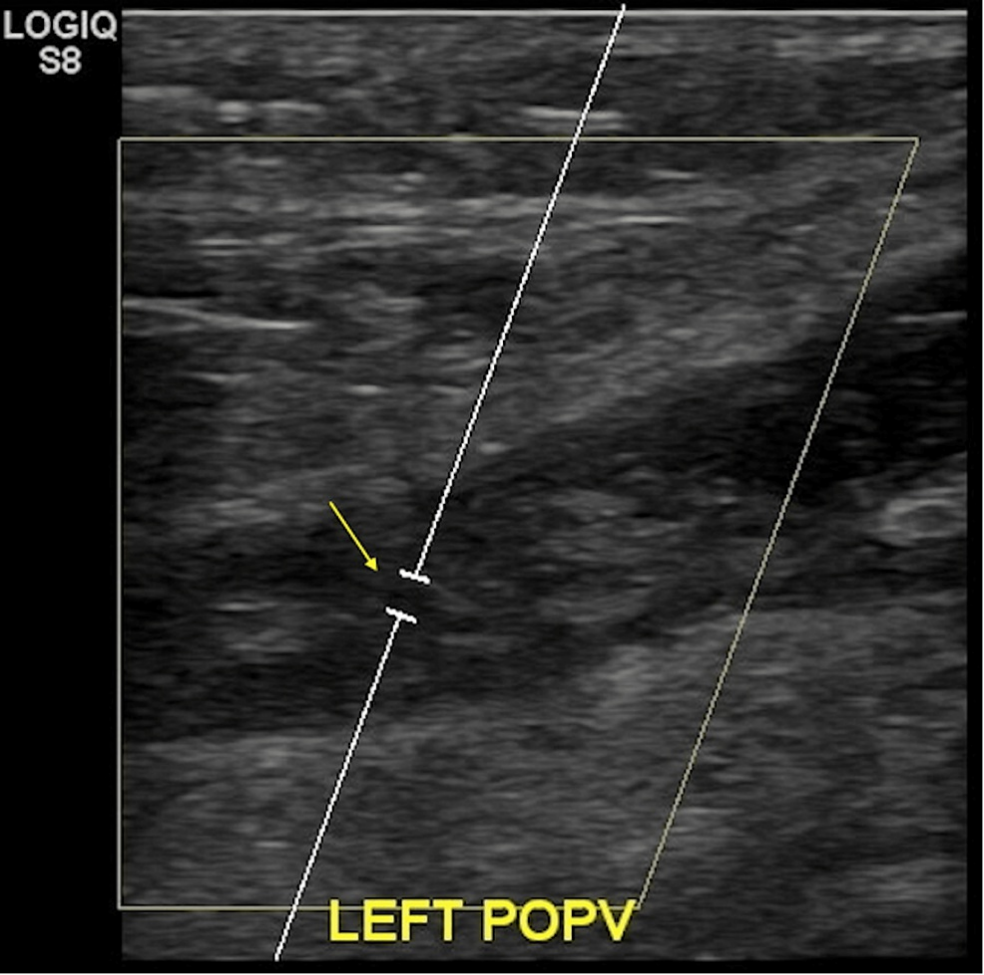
Imaging of patient’s lower left extremity reveals deep vein thrombosis as assessed by Doppler ultrasonography
